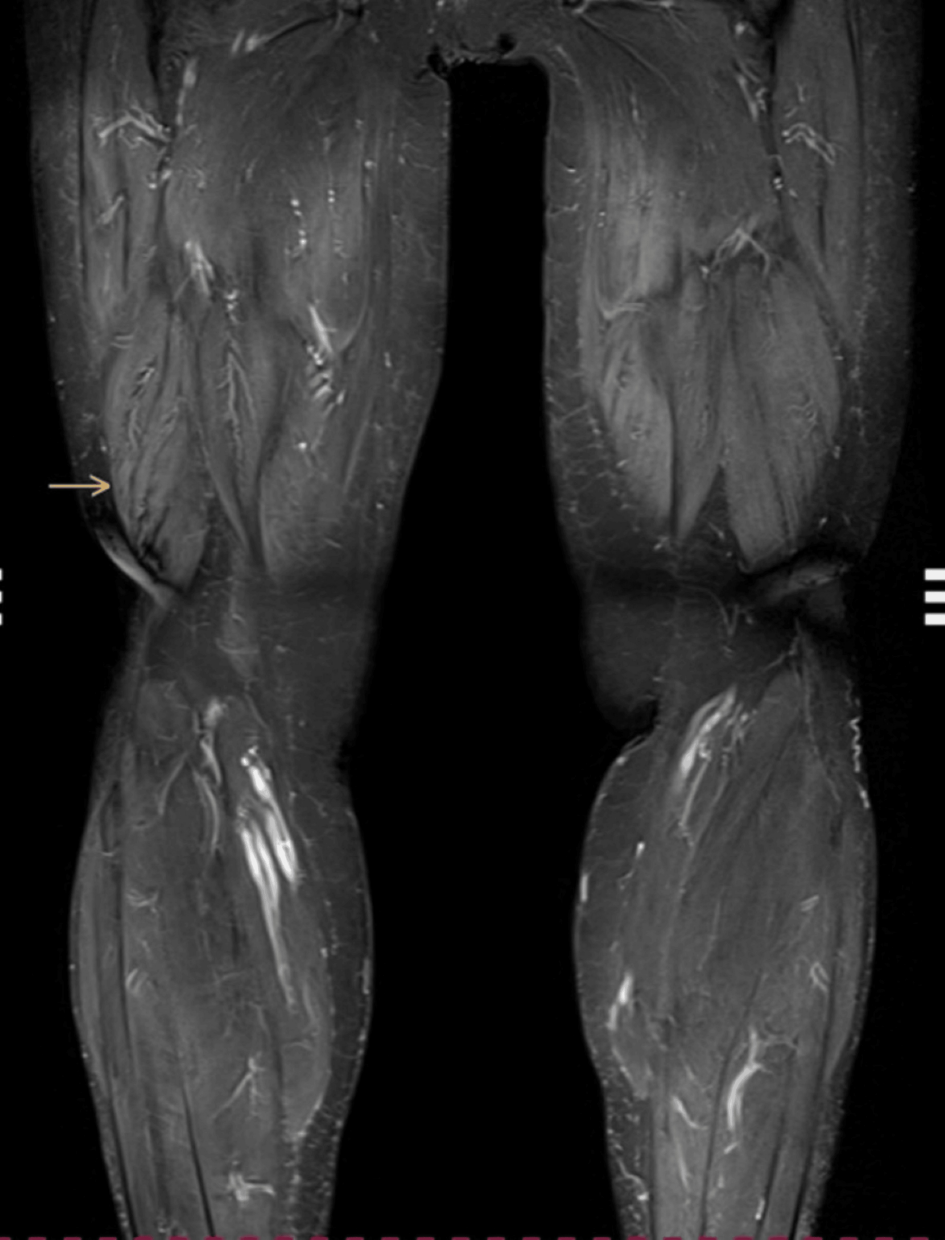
MRI of the legs The yellow arrow indicates an altered T2 signal in multiple muscles, consistent with myositic changes.
